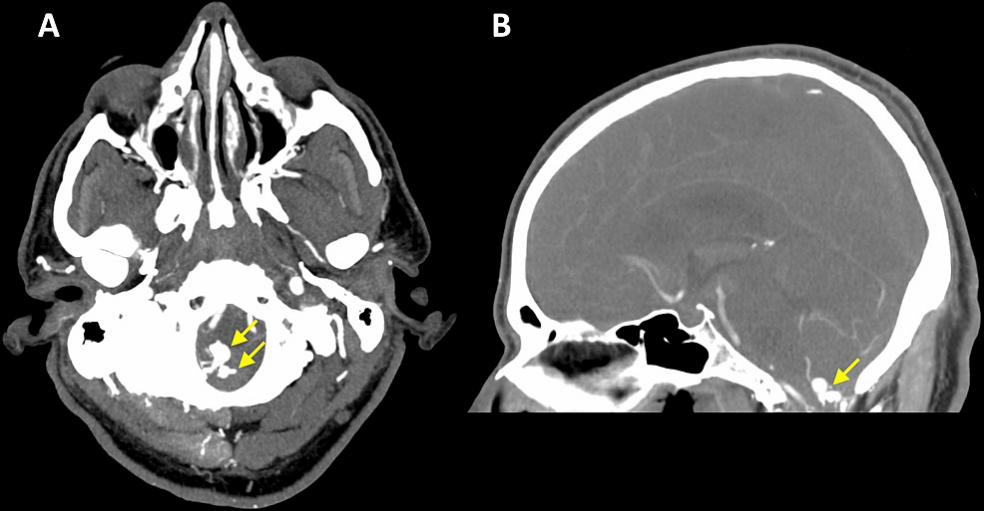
(A) Transverse CT angiogram demonstrating AVM nidus (arrow) within the foramen magnum. (B) Sagittal CT angiogram demonstrating AVM nidus (arrow) within the foramen magnum with communicating vasculature visualized. CT: computed tomography; AVM: arteriovenous malformation.
